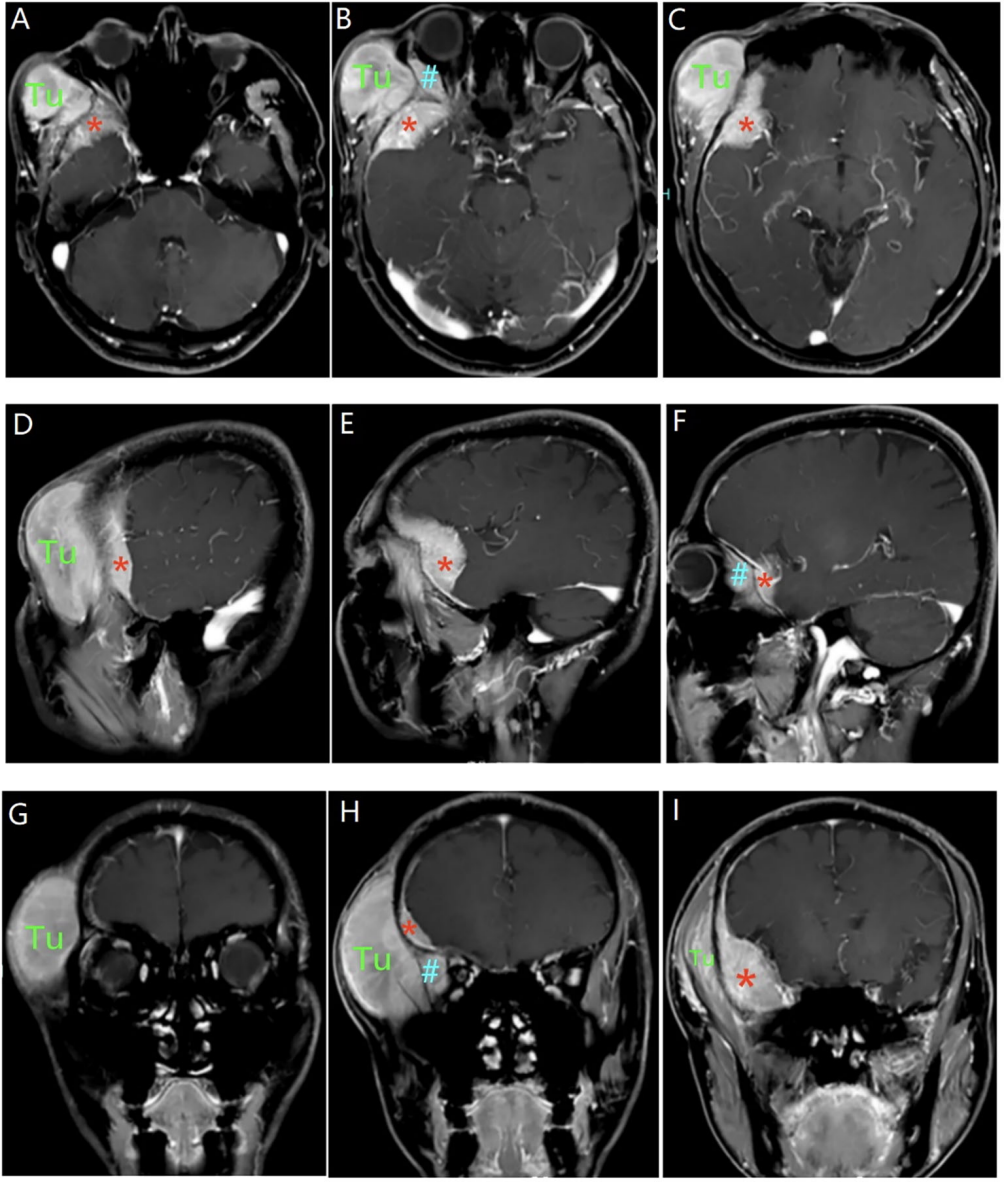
Preoperative post-contrast T1-weighted MRI in multiple planes. Representative post-gadolinium T1-weighted MRI shows homogeneously enhancing lesions involving the right frontotemporal subcutaneous tissue (Tu), orbit (*), and the floor of the middle cranial fossa (#) on axial (A–C), sagittal (D–F), and coronal (G–I) images. Tu, tumor; *, intraorbital lesion; #, middle cranial fossa lesion.
